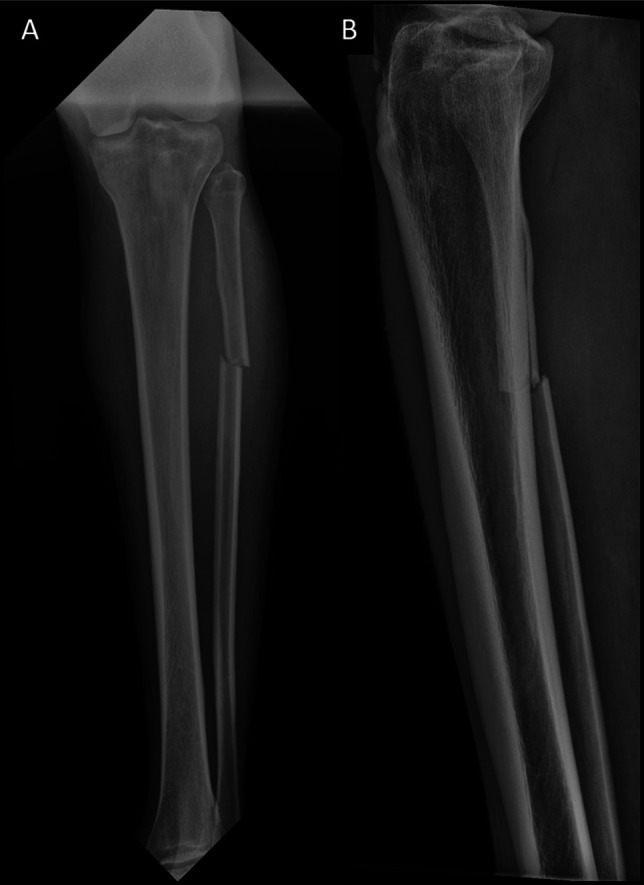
Anteroposterior and lateral radiographs of the left tibia and fibula demonstrating isolated fibular shaft fracture on the day of presentation. Fracture is less than 50% displaced in both planes although it is located in an area of higher soft-tissue volume.
